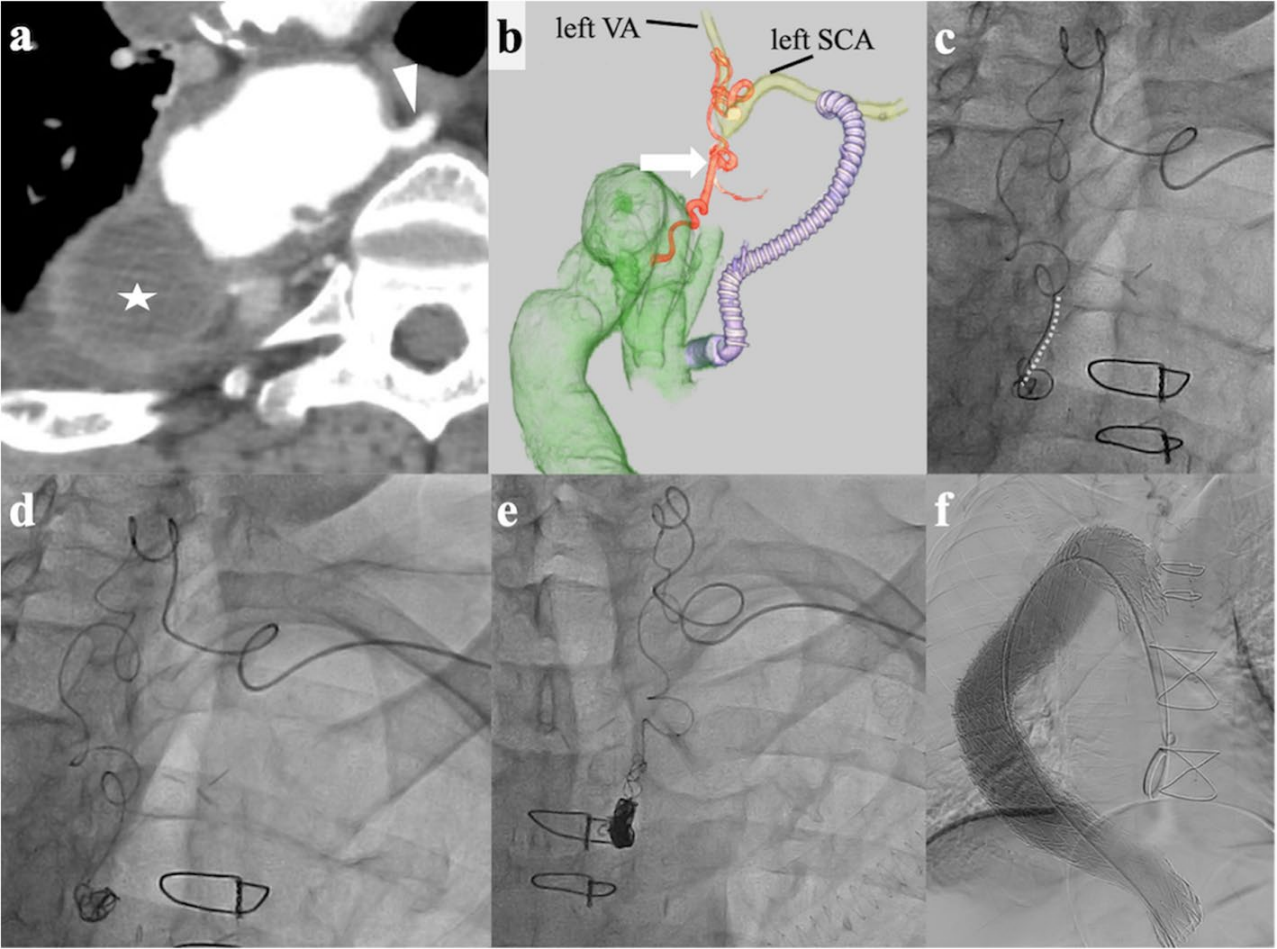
A 69-year-old male presented with an extensive thoracic aortic aneurysm, a right aortic arch, and an isolated left subclavian artery (SCA). Initially, we performed an elephant trunk ascending aorta replacement and reconstructed the cervical branches. Subsequently, we planned for thoracic endovascular aortic repair (TEVAR) to address the aortic arch and descending aortic aneurysm. a contrast-enhanced computed tomography revealed a dilated third intercostal artery measuring 4 mm (arrowhead) and a coarse mural thrombus within the aneurysm (star). Embolization of the artery was planned to prevent a type 2 endoleak; b a three-dimensional volume-rendering image demonstrated that the highly tortuous third intercostal artery (arrow) anastomosed with the left costocervical trunk, which arises from the left SCA, and that the left vertebral artery (VA) branched off closely to the left costocervical trunk. Considering the risk of peripheral embolization of the VA due to a substantial mural thrombus, we punctured the left brachial artery and accessed this artery from the left SCA; c a 1.6-Fr microcatheter (Carnelian Marvel S; Tokai Medical Products, Aichi, Japan) was advanced near the origin of the third intercostal artery, and we attempted to place a 0.014-inch coil (Target XL soft coil; Stryker, Fremont, CA, USA) measuring 5 mm in diameter and 10 cm in length. However, the pathway’s tortuosity was so severe that we could not implant the entire coil, and half of it remained within the catheter (dotted line); d therefore, the coil was intentionally detached within the catheter and flushed with saline solution for placement; e seven additional 0.014-inch coils (six target XL soft coils and one Azur soft 3D coil; Terumo, Tokyo, Japan) were tightly placed using the intentional early detachment technique; f final angiogram after TEVAR shows successful aortic repair without any endoleaks
